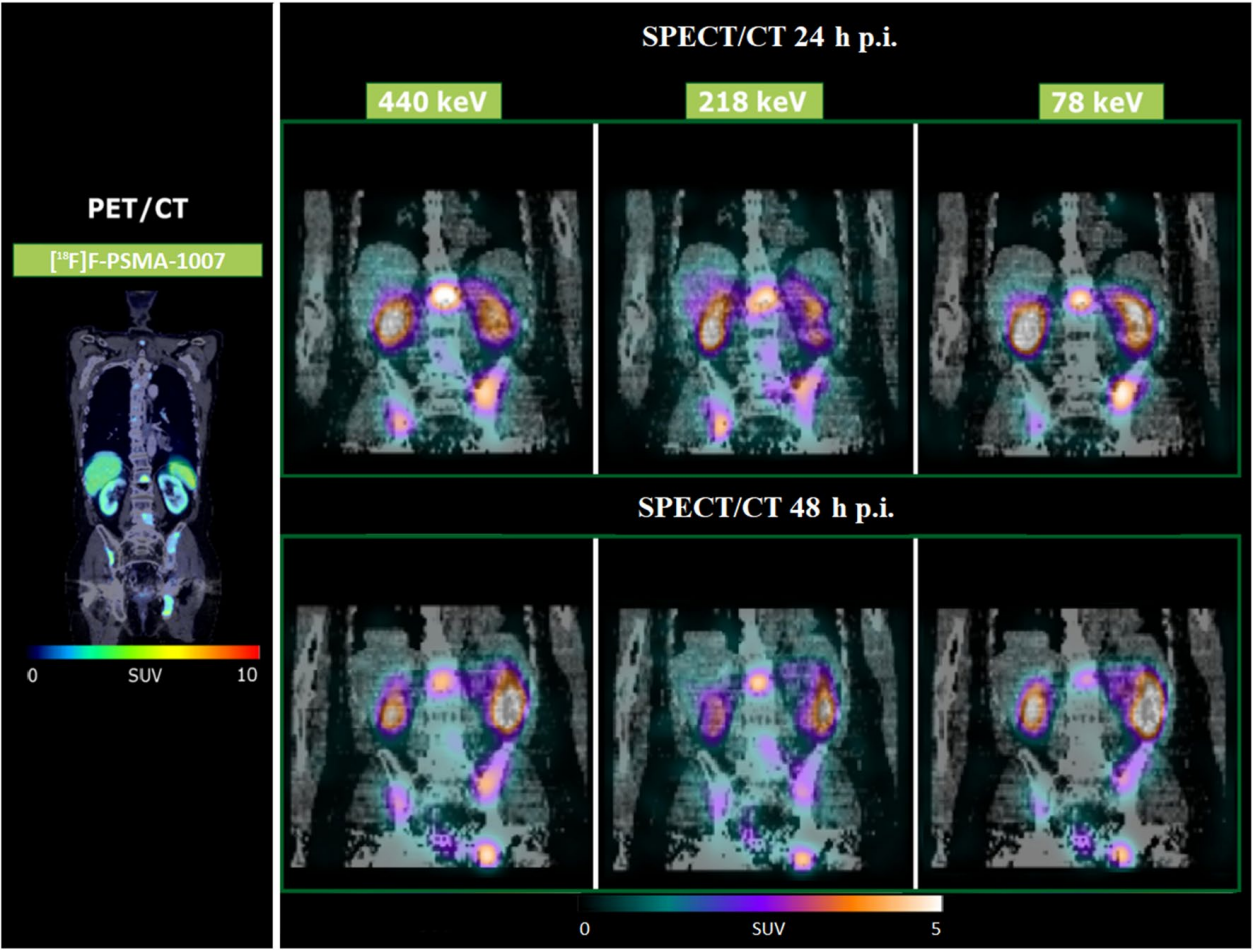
Pre-therapy PET/CT and post-filtered SPECT/CT acquired at 24 and 48 h post-treatment (Gaussian post-filter, FWHM 30 mm) for patient 3
